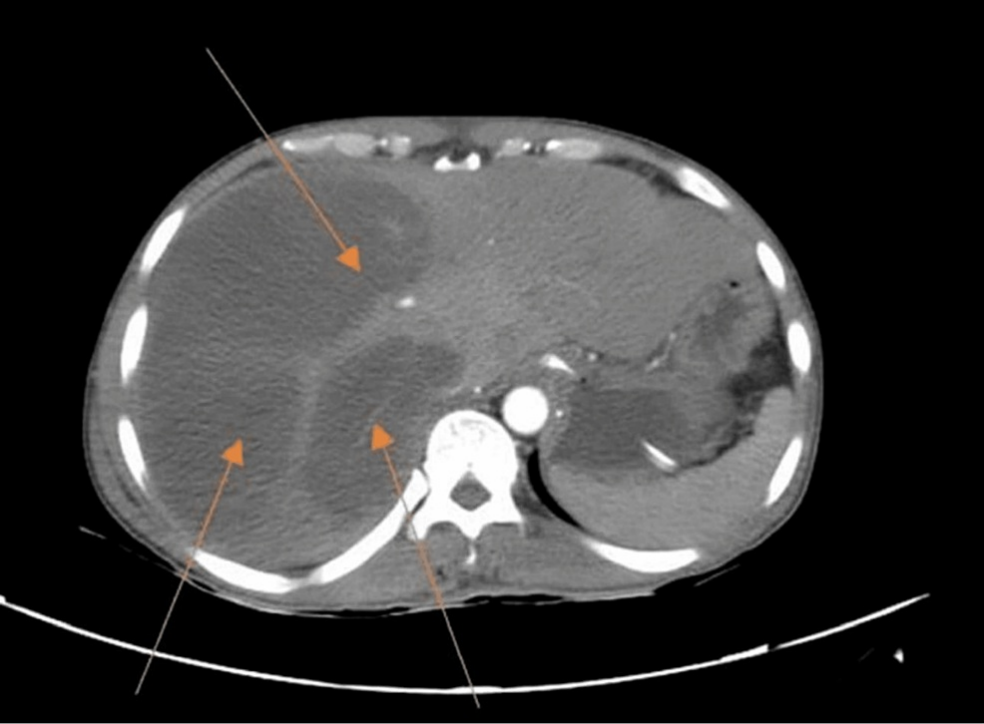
CT scan with IV contrast showing multiple liver abscesses (arrows) CT: computed tomography
